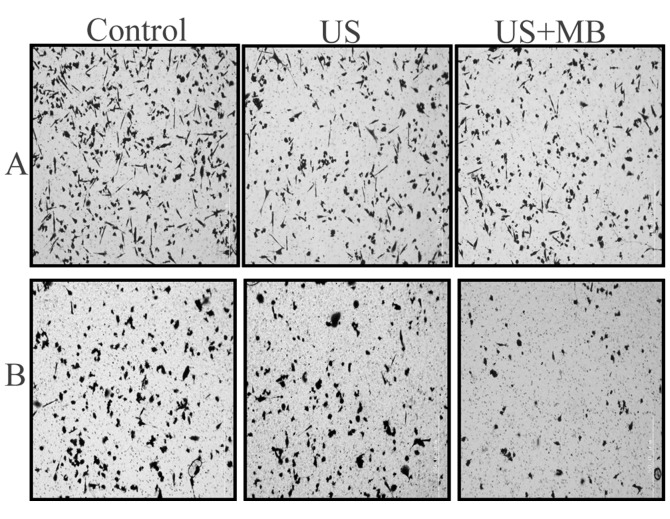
Ultrasound treatment combined with microbubbles suppresses the (A) migration and (B) invasion of PC-3 cells. US, ultrasound group; US + MB, ultrasound in combination with microbubbles group (magnification, ×200).
